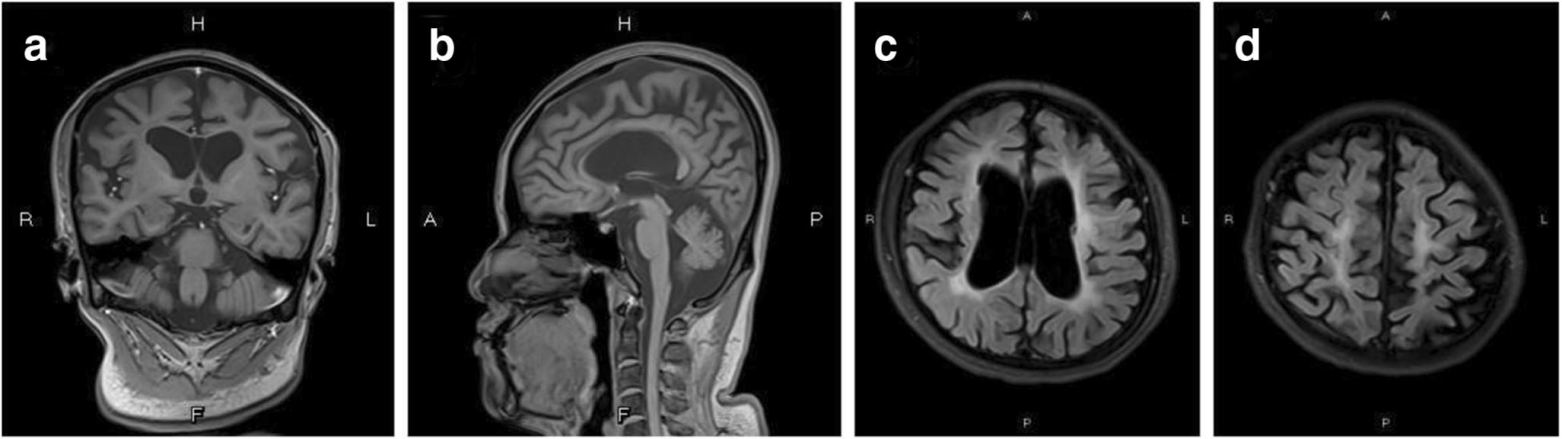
MRI. Neuroimaging of patient III-1. a T1-weighted coronal MRI shows diffuse brain atrophy with a frontotemporal predominance and enlargement of the ventricles. b T1-weighted sagittal MRI shows brain atrophy and severe thinning of the corpus callosum. c FLAIR axial MRI shows brain atrophy, ventriculomegaly and symmetrical hyperintensity of the periventricular white matter. d FLAIR axial MRI shows subcortical bifrontal and frontoparietal cerebral white matter hyperintensity
